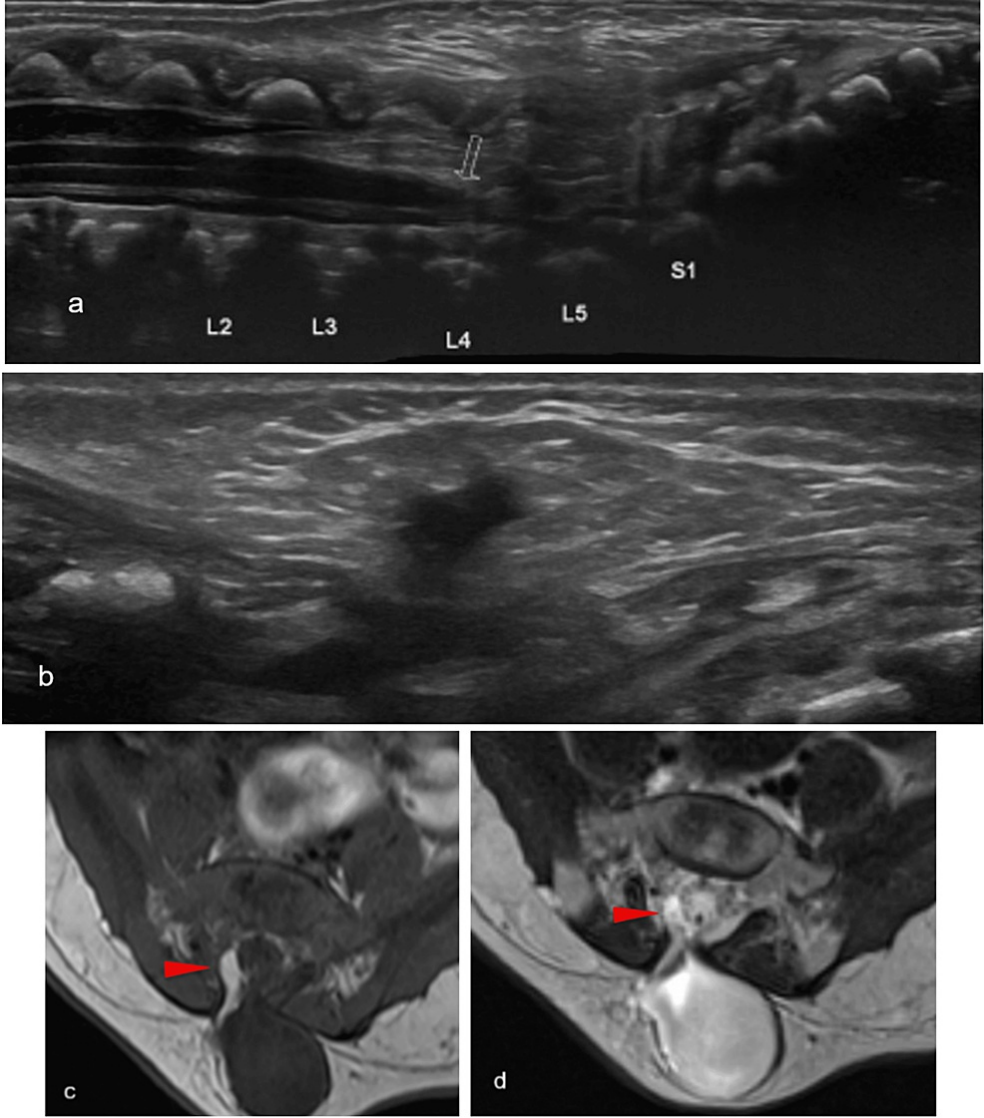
Spinal lipoma Longitudinal (a) and transverse (b) ultrasound images showing the cystic structure in soft tissues overlying L4-5. T1 (c) and T2 (d) MR images in the axial plane depicting a T1 hyperintense component, in keeping with fat Radiological images obtained by the authors
